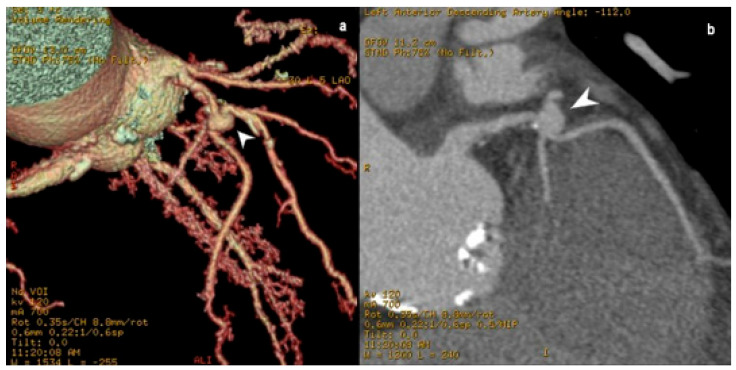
MD CT coronary angiography three-dimensional-volume rendered image (a) and planar view (b) images, showing a left anterior descending coronary artery saccular aneurysm (white arrowheads). The presented patient was managed conservatively, with radiological follow-up to monitor for aneurysmal enlargement, and was eventually referred to referent healthcare institution for surgical treatment.
